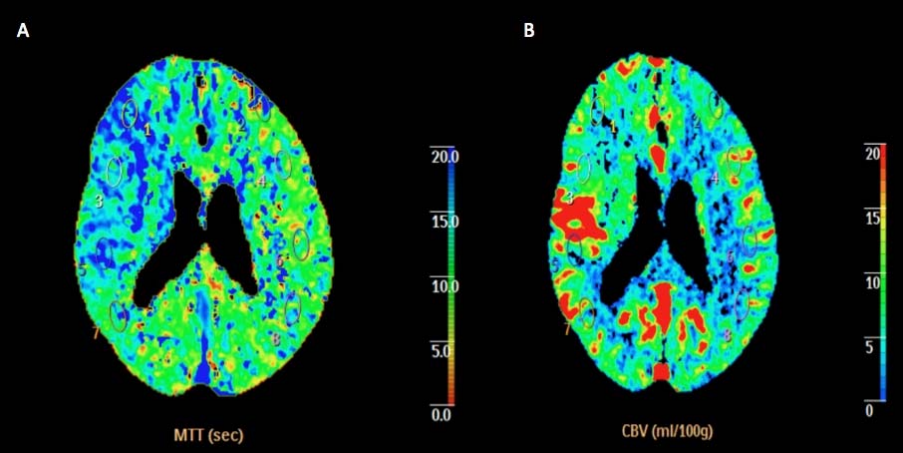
Computed tomography perfusion scan of the brain shows (A) increased mean transit times (MTT) in right middle cerebral artery territory and (B) normal symmetric cerebral blood volumes (CBV), indicative of a large penumbra.
